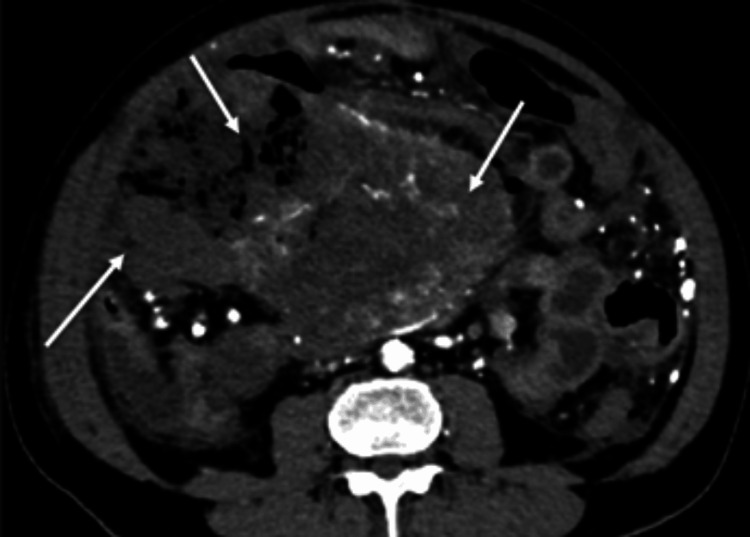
Axial CECT image in arterial phase showing a large lobulated heterogeneously hyperenhancing mass with central necrosis in the right lower quadrant (white arrows) CECT: contrast-enhanced computed tomography
